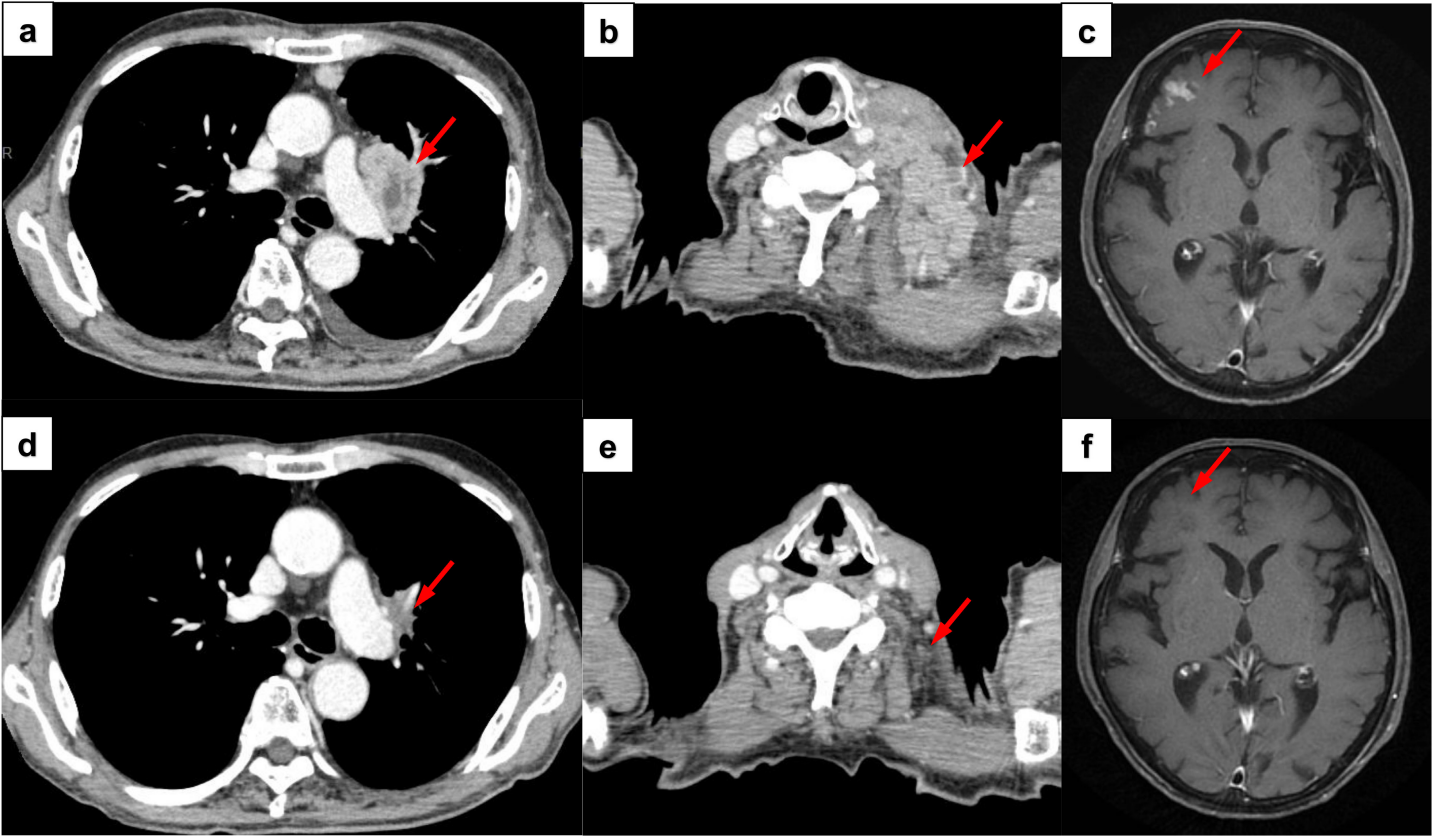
Imaging findings of the present case before and after two cycles of chemotherapy. Contrast‐enhanced computed tomography (CECT) scans before chemotherapy showed (a) lung cancer in the left upper lobe and (b) metastases to the left supraclavicular lymph nodes. (c) Contrast‐enhanced magnetic resonance imaging (MRI) showed brain metastases in the right frontal lobe. Images (d–f) represent the corresponding findings after two cycles of chemotherapy.
